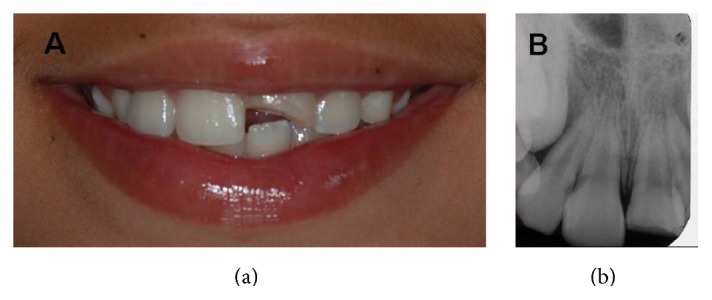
(a) Initial clinical aspect. (b) Initial radiographic aspect.
